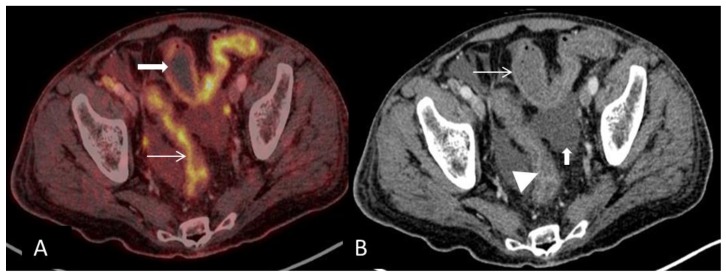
(A) Axial PET and CT fused image shows pathological high FDG-uptake in the large-bowel in two different patterns corresponding to the lumen (arrow) and in the outer wall (bold arrow) respectively and is consistent with an inflammatory response. (B) CT scan shows mural stratification (i.e., visualization of two or three different layers of the bowel wall) of the thickened large-bowel wall (defined as >3 mm) with mucosal (arrowhead) and serosal enhancement (arrow). The PET/CT findings described here are typical for acute GVHD-GIT or inflammatory bowel disease. Ascites is a common finding in acute-GVHD and has been reported to occur in 45% of affected patients (bold arrow) [2].
